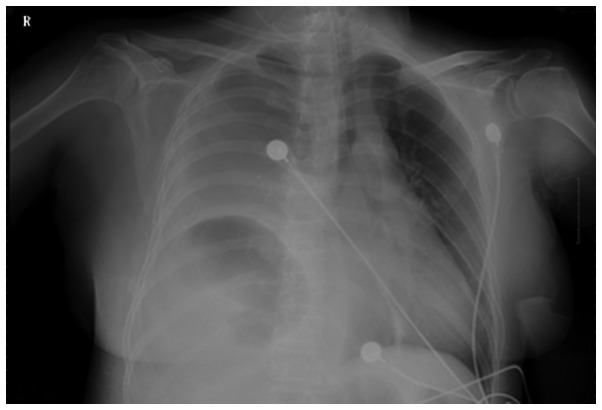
Chest X-ray shows a right pleural effusion and enlarged bowel in the chest cavity.
